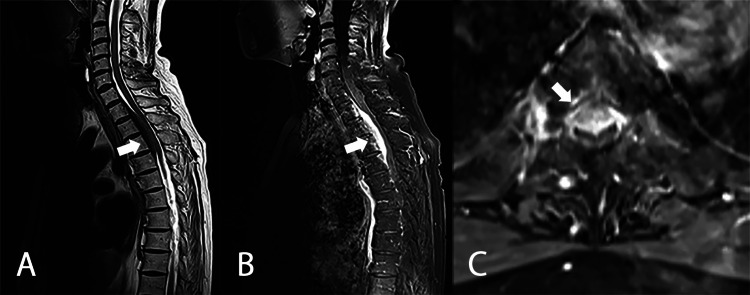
Initial thoracic MRI of case 1 A: T2WI sagittal; B: T1WI contrast enhancement sagittal; C: T1WI contrast enhancement axial. Extramedullary mass lesion located ventral to the spinal cord in T1-T4 vertebral level. The initial radiological diagnosis was a meningioma MRI: magnetic resonance imaging
